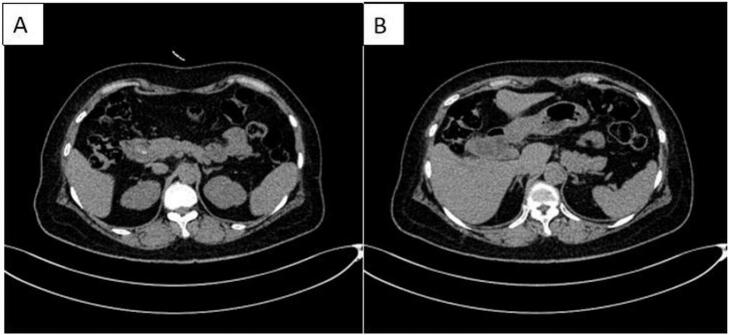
Preoperative CT A:The common bile duct is obstructed by a stone and dilated, with slight thickening of the lower end wall; B:bile stasis and cholecystitis, with the possibility of gallbladder neck stones.
